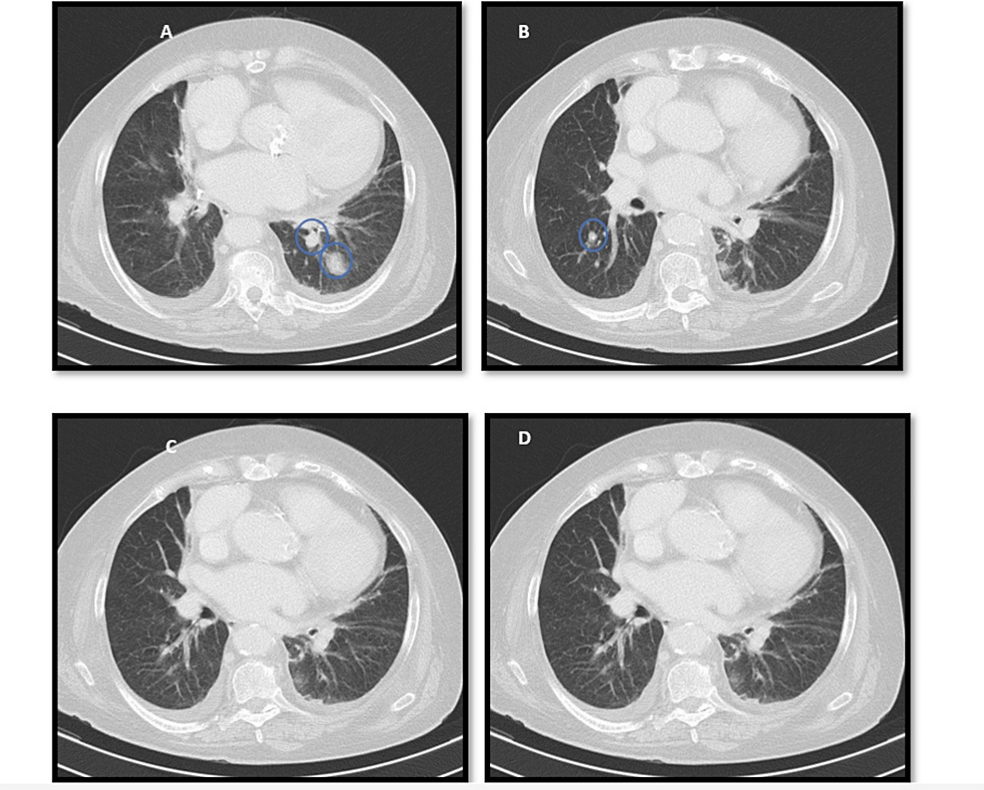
First (A, B) and second (C, D) computed tomography scans. (A, B) Three lesions in the lungs and (C, D) lesions had disappeared.
